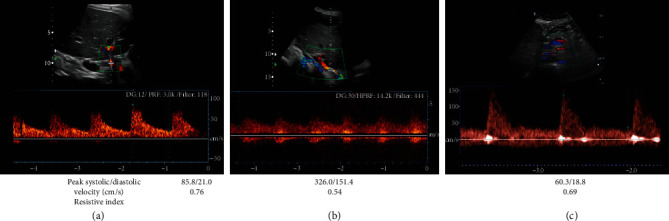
Case 1 baseline Doppler ultrasound with appropriate posttransplant waveforms at the recipient left gastric to graft artery anastomosis (a). Dampened “parvus tardus” waveforms concerning for arterial stenosis at the anastomosis on postoperative day 37 prior to intervention. Appropriate arterial waveforms three-year posttransplant after successful primary balloon angioplasty (c).
